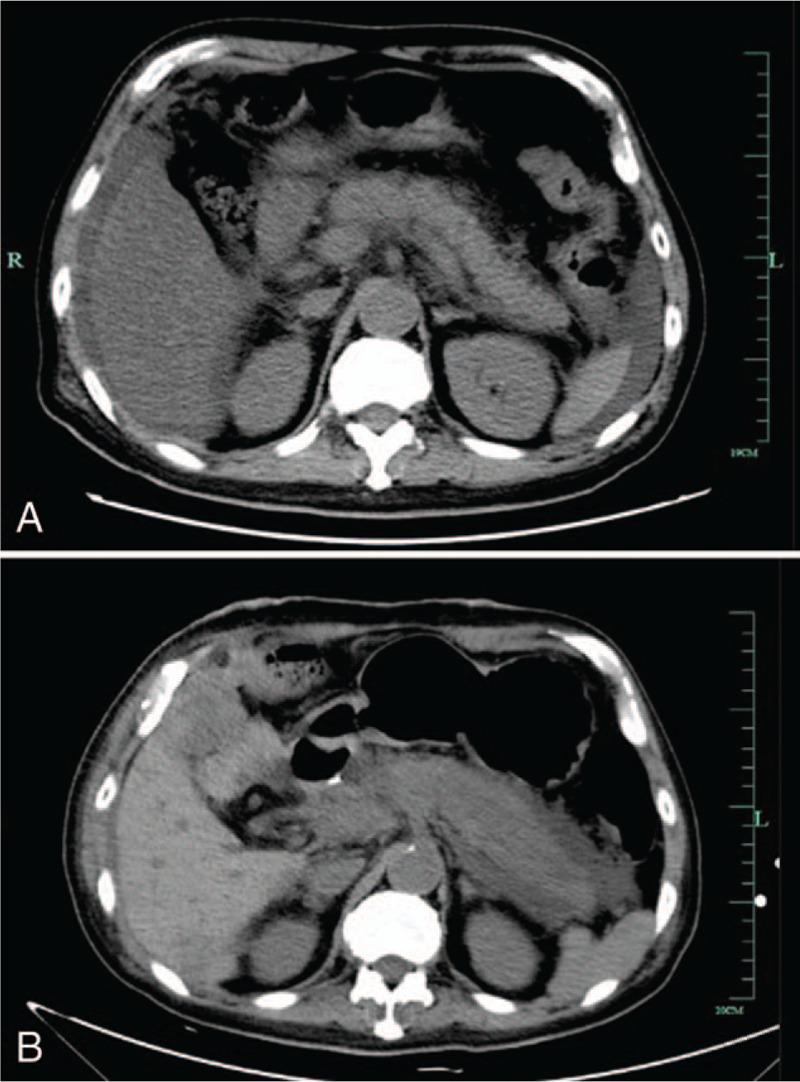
(A) Before surgery, abdominal computed tomography (CT) showed pelvic and peritoneal cavity fluids and an unclear margin of the pancreas. (B) After surgery, abdominal CT showed pancreatic swelling with massive exudation and effusion.
